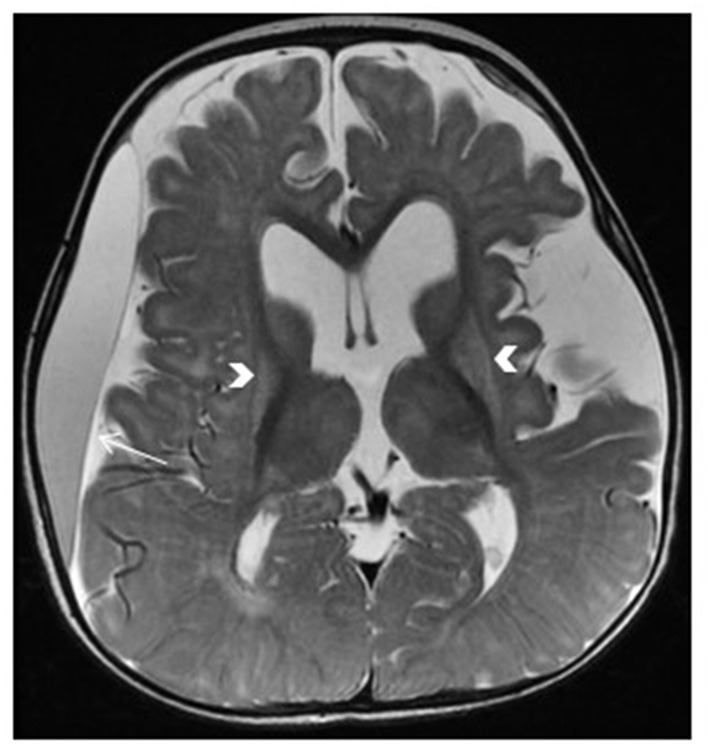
Glutaric aciduria type 1. Brain MRI: axial T2-Weighted image demonstrates bilateral abnormal hyperintense signal and atrophic changes of the putamina (arrows), enlarged subarachnoid spaces, dilated lateral ventricles, and subdural effusion predominantly on the right side (arrow).
